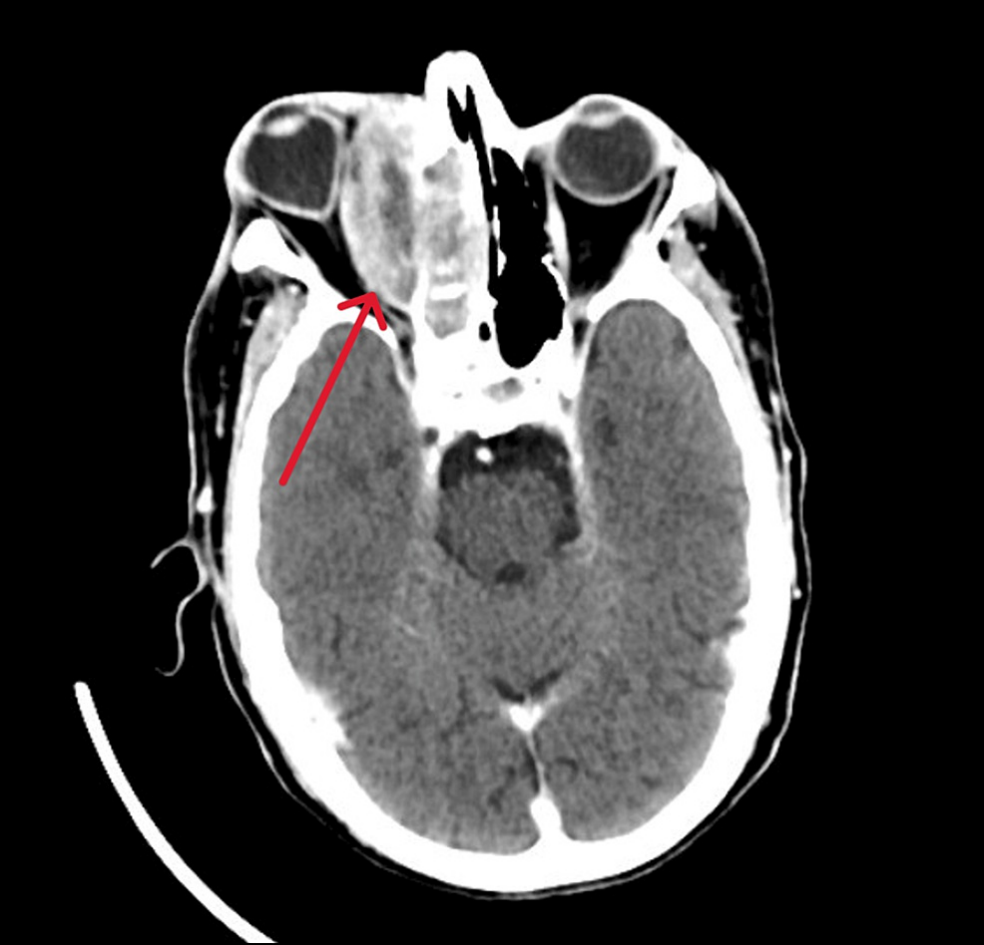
Case 2: Axial view CT shows the mass from the right nasal cavity extending into the orbital space (arrow). Noted also was a mass effect toward the orbit.
